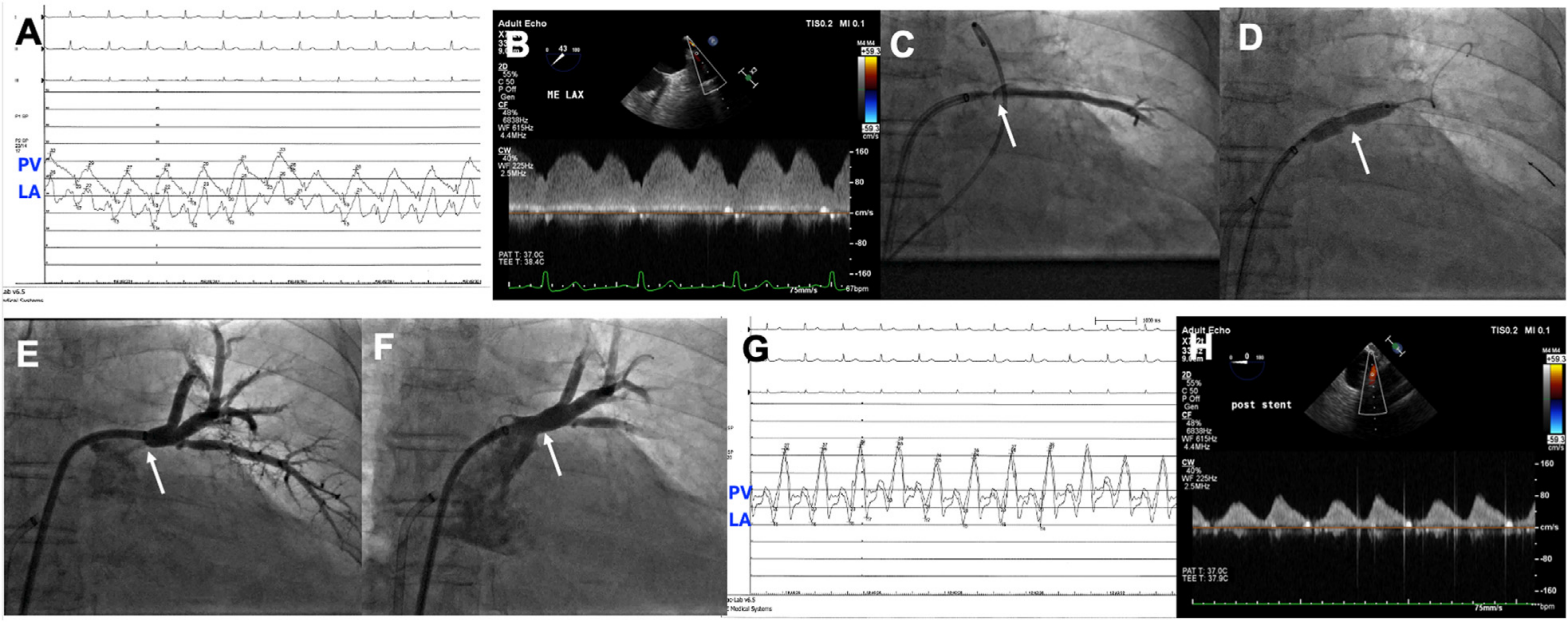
Pulmonary vein (PV) stenosis evaluation and intervention. (A) Simultaneous pressure in the PV (loss of the typical phasic flow) and the left atrium (LA), (B) left upper lobe pulmonary vein (LUPV) Doppler flow characteristic preintervention, (C, D) ballooning of the LUPV, (E) restenosis of the PV ostium after ballooning, (F) poststenting of LUPV ostium, (G) simultaneous pressure in PV and LA, poststenting, and (H) LUPV Doppler flow characteristic poststenting.
